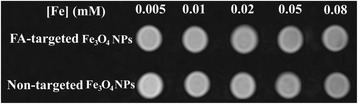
The T_2_-weighted MR images of the FA-targeted Fe_3_O_4_ NPs and non-targeted Fe_3_O_4_ NPs at different Fe concentrations
